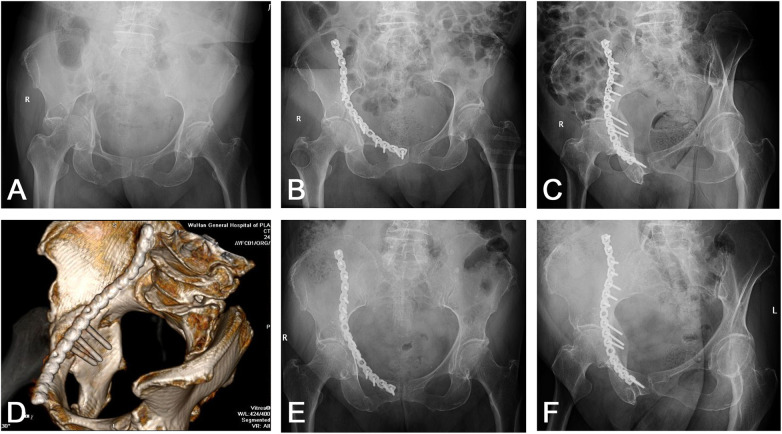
Case of a 67-year-old woman had a transverse acetabular fracture and superior pubic ramus fractures. (A) Preoperative AP view x-ray shows a protrusion of the femoral head. (B) Postoperative AP and (C) iliac oblique view radiograph shows a satisfactory reduction of the QLP fractures. (D) Postoperative CT scan with 3D reconstruction demonstrating three quadrilateral screws were placed parallel to the quadrilateral surface. (E,F) Postoperative AP and iliac oblique views x-rays of the pelvis at 4 years after the operation showing an excellent radiological outcome, and the patient was symptom free.
